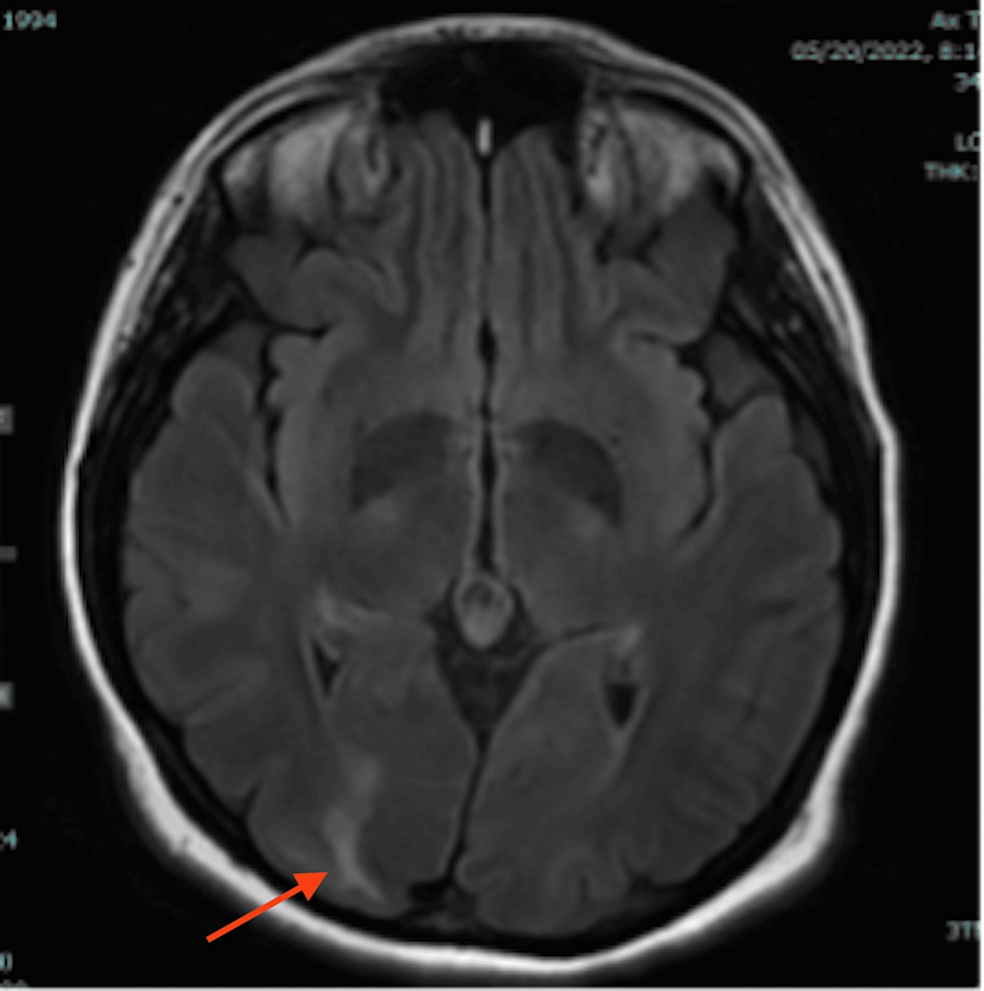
T2/FLAIR MRI of the brain (image I) FLAIR: Fluid attenuated inversion recovery The red arrow shows hyperintensity in the right occipital lobe.
